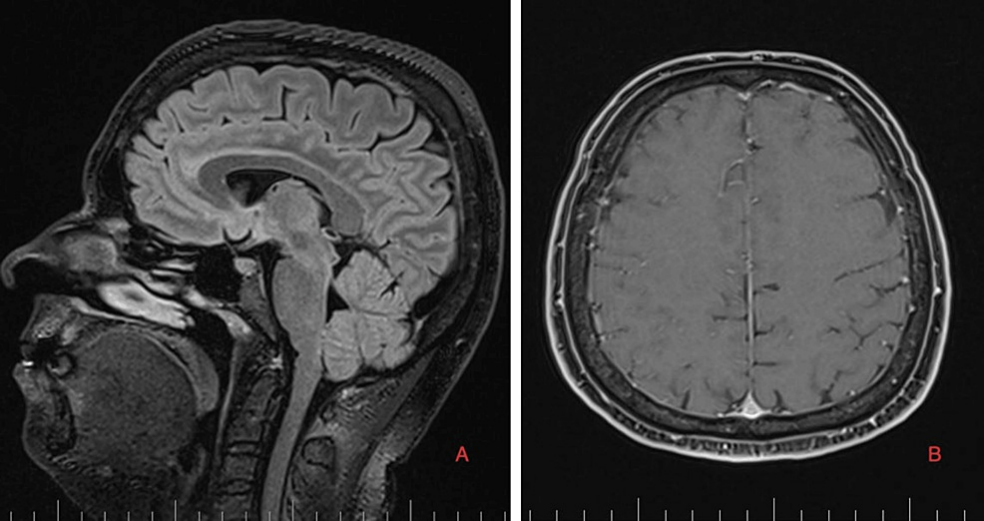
Brain MRI on September 4, 2023. A: Sagittal T1 with contrast images showing total regression of spontaneous intracranial hypotension signs. B: Axial T1 with contrast images without any pachymeningeal enhancement.
